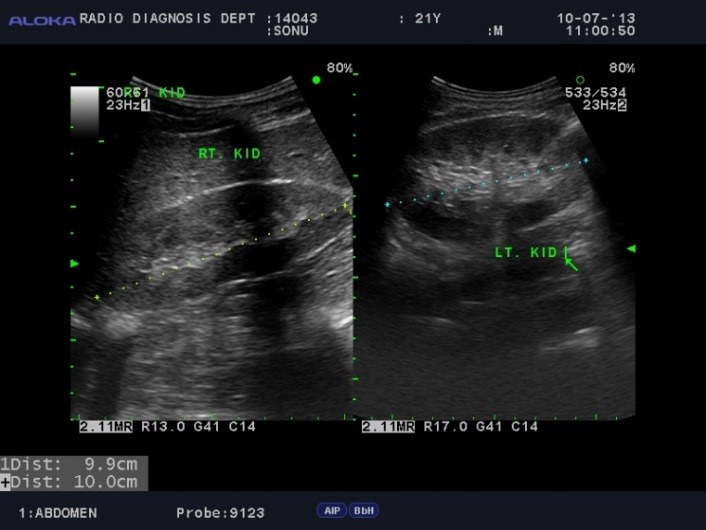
Ultrasonography of right kidney showing hypoechoic areas suggestive of nephritis
